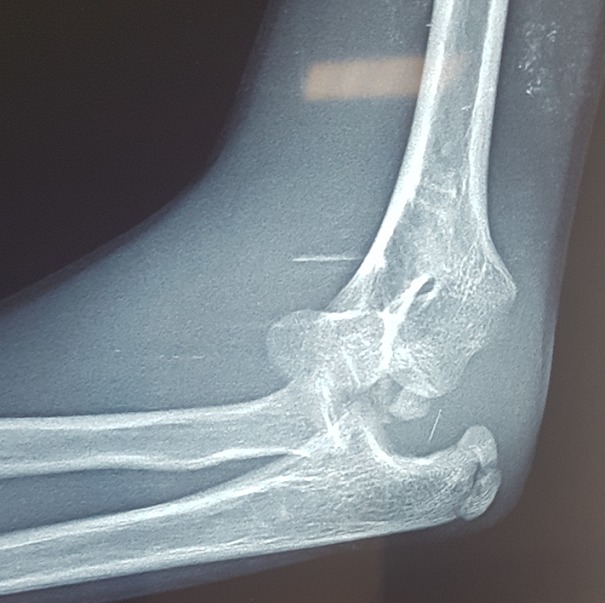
Image d’incarcération de l’épicondyle médial sur la radiographie de profil
